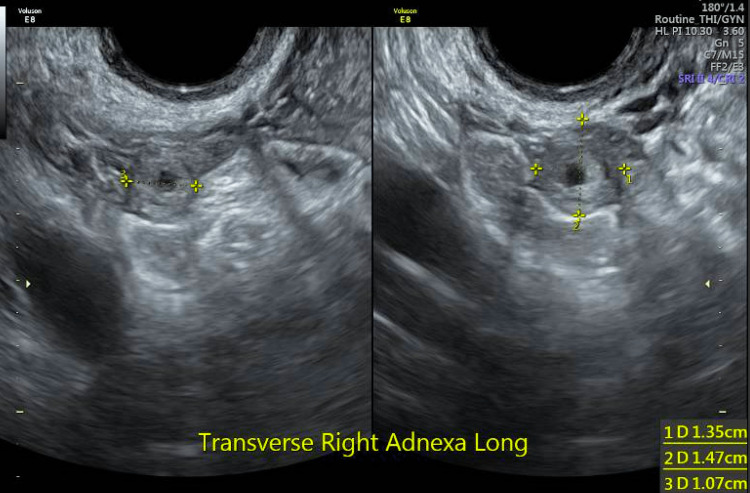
Right adnexal mass on transvaginal ultrasound
